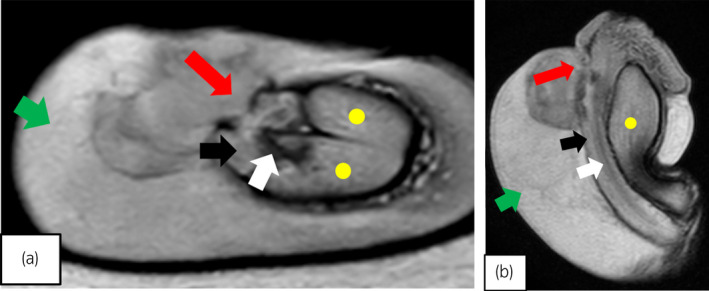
T2‐weighted magnetic resonance imaging shows an injury of the ventral tunica albuginea of the corpus spongiosum. (a) axial image. (b) sagital image. Green arrows: hematoma; red arrows: rupture of the corpus spongiosum; black arrows: corpus spongiosum; Yellow dots: corpus cavernosum; white arrows: urethra.
